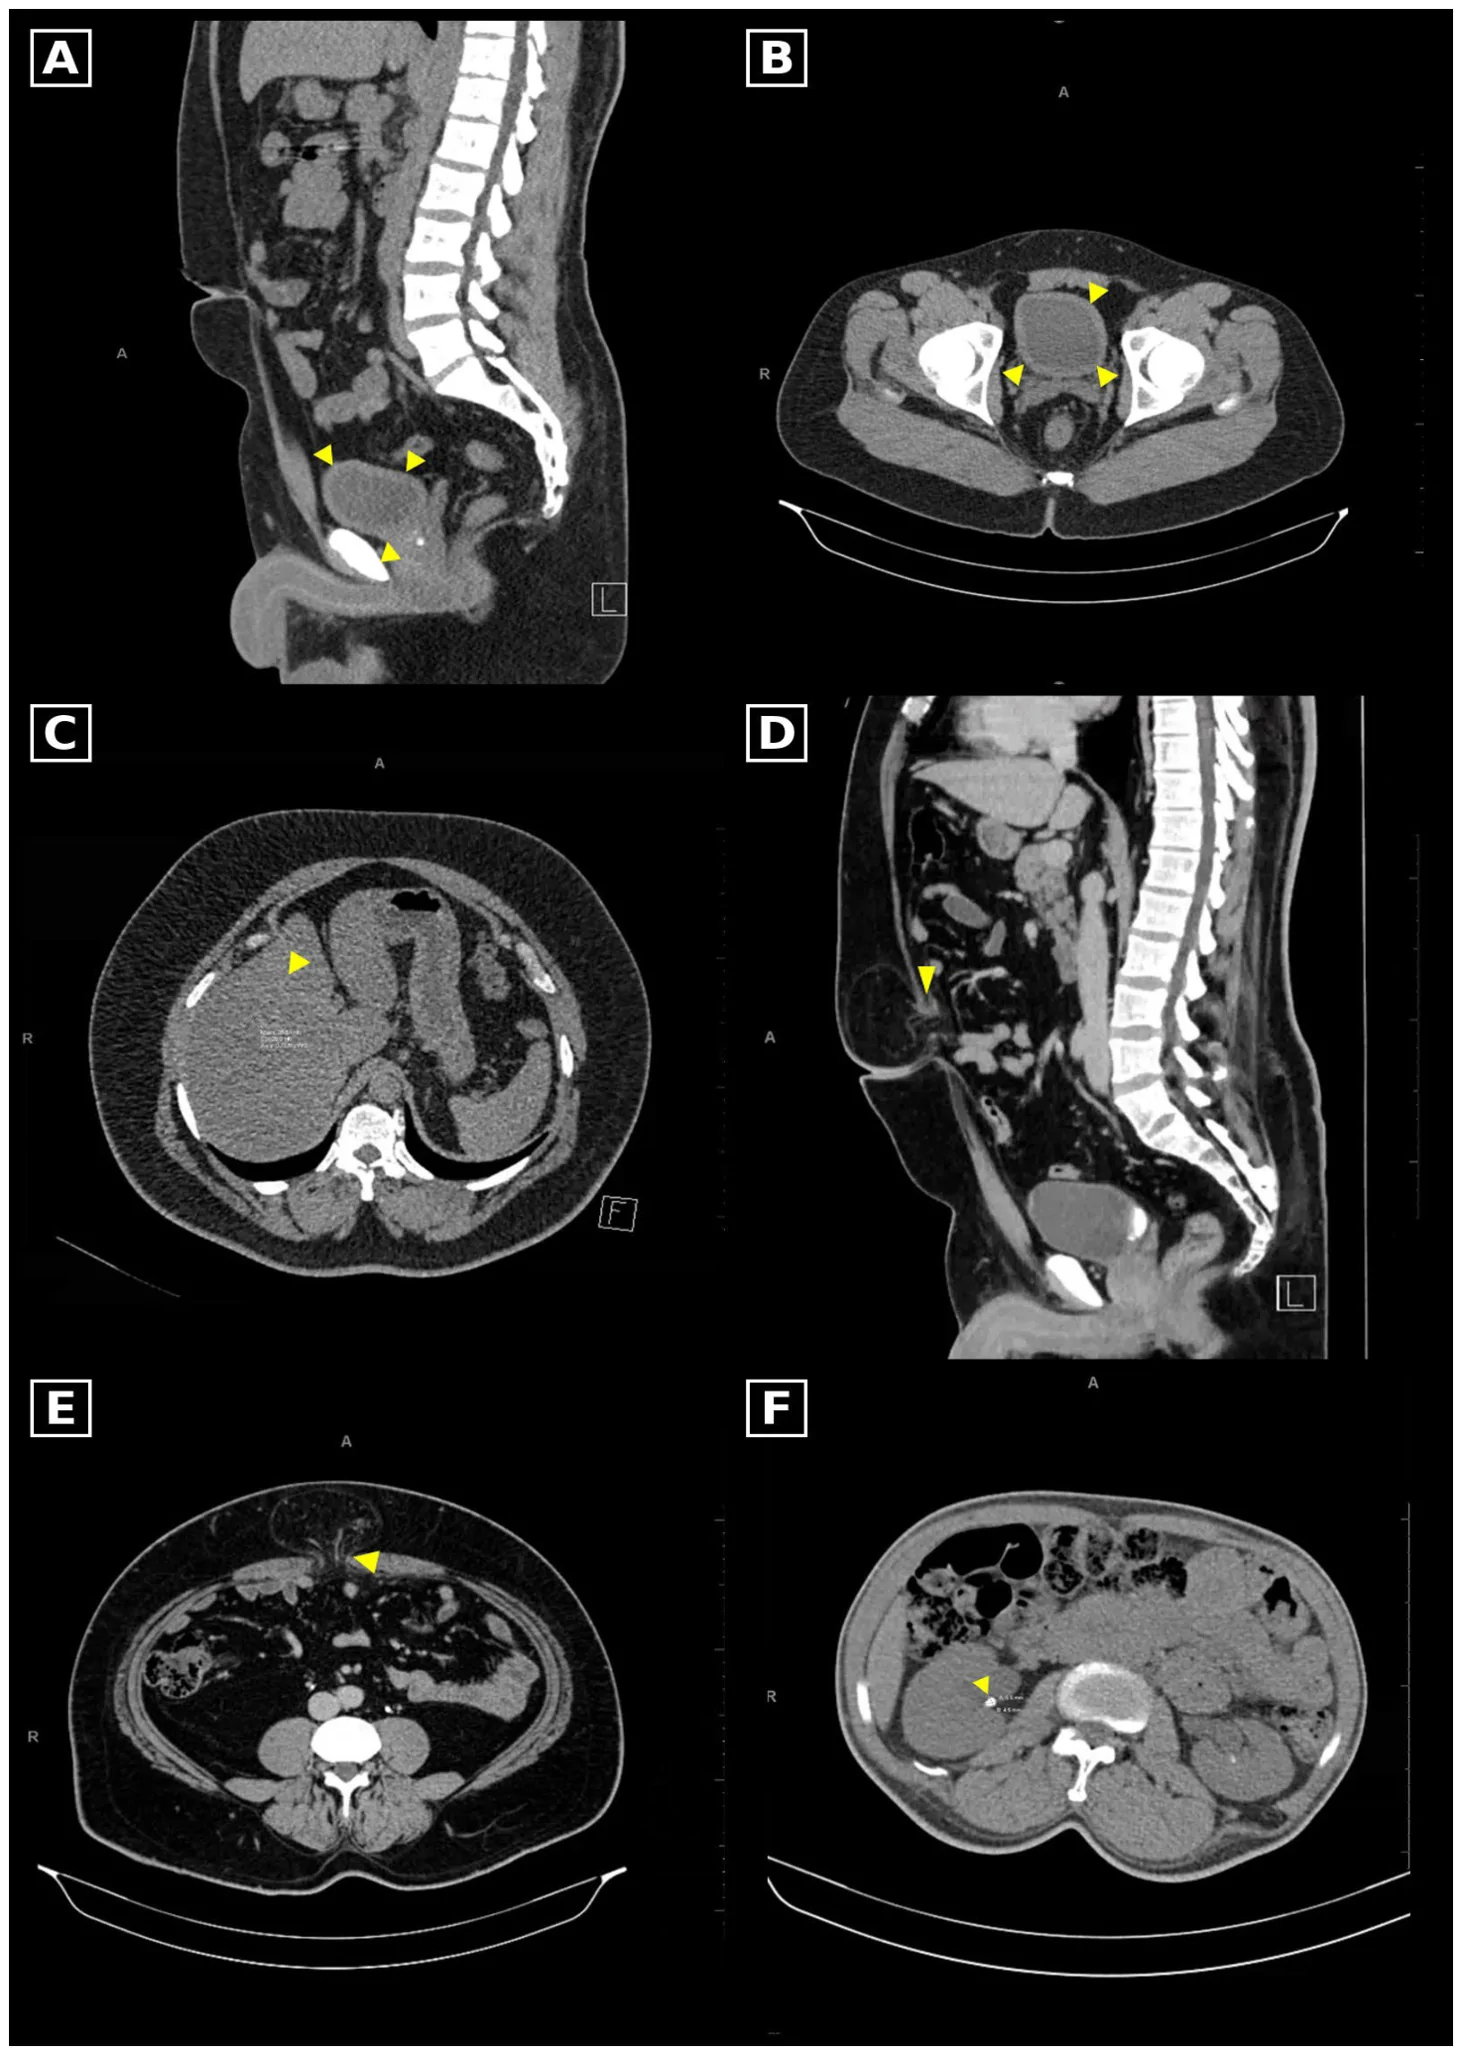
Representative CT images of selected incidental findings identified in the study. (A,B) Mild diffuse circumferential urinary bladder wall thickening in a 29-year-old man on sagittal and axial non-contrast CT images, respectively. (C) Diffusely decreased hepatic attenuation consistent with hepatic steatosis in a 42-year-old man on axial non-contrast CT. (D,E) Supraumbilical fat-containing hernia in a 43-year-old man on sagittal and axial contrast-enhanced CT images, respectively. (F) Right renal pelvic calculus measuring approximately 6 × 5 × 8 mm with an attenuation of 1340 HU in a 51-year-old man on axial non-contrast CT. Arrowheads indicate the relevant incidental findings.
